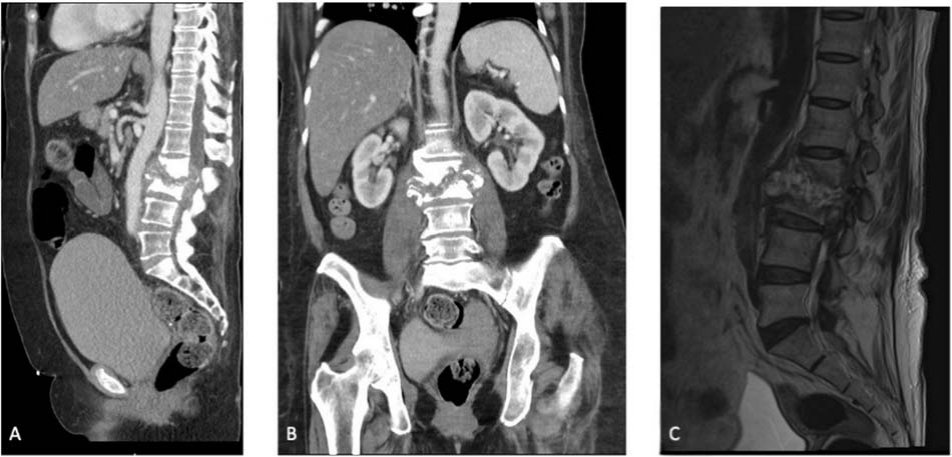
(A) Sagittal section of a CT scan depicting L2–L3 discitis-osteomyelitis. (B) Coronal section of a CT scan depicting L2–L3 discitis-osteomyelitis. (C) Sagittal MRI of the lumbar spine demonstrating severe L2–L3 discitis and L2–L3 osteomyelitis with a large anterior epidural abscess causing severe spinal stenosis at the L2 and L3 levels.
